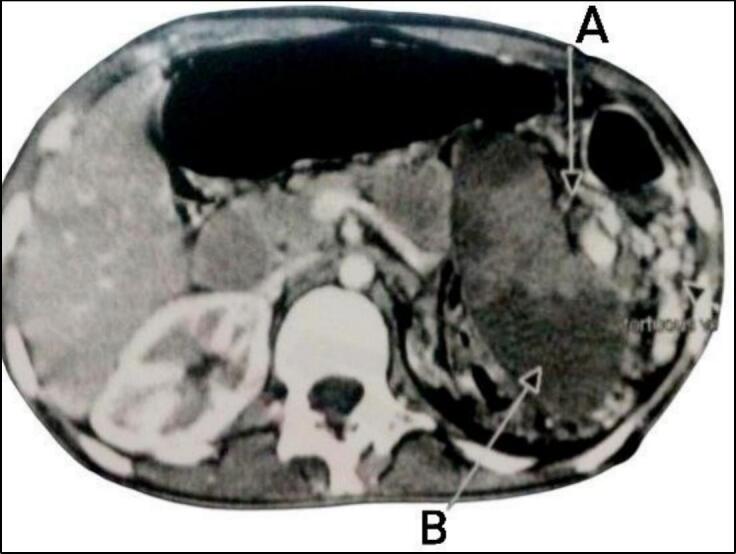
Selected cut of contrast-enhanced CT of the abdomen showing an enlarged spleen with torsion of its pedicle (A) and heterogeneous hypodense areas that consistent with infarction (B).
